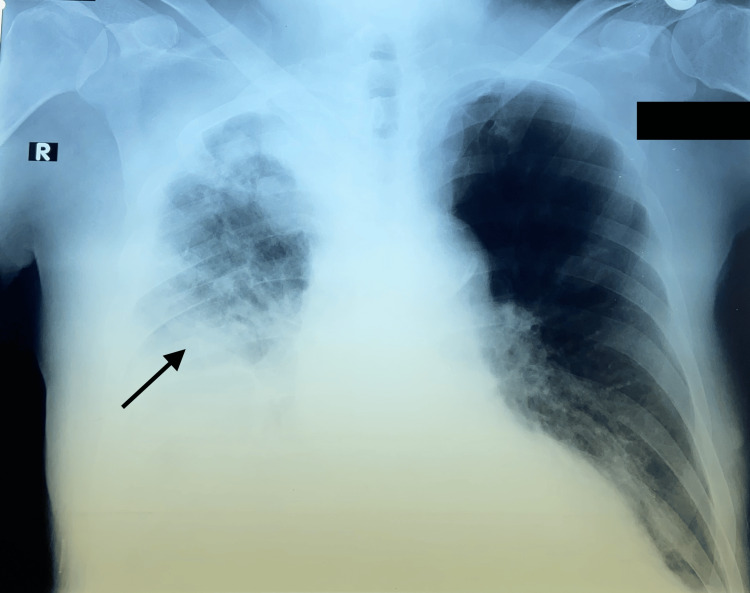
Anterior-posterior view chest X-ray showing right-sided pneumonia with pleural effusion (black arrow).
